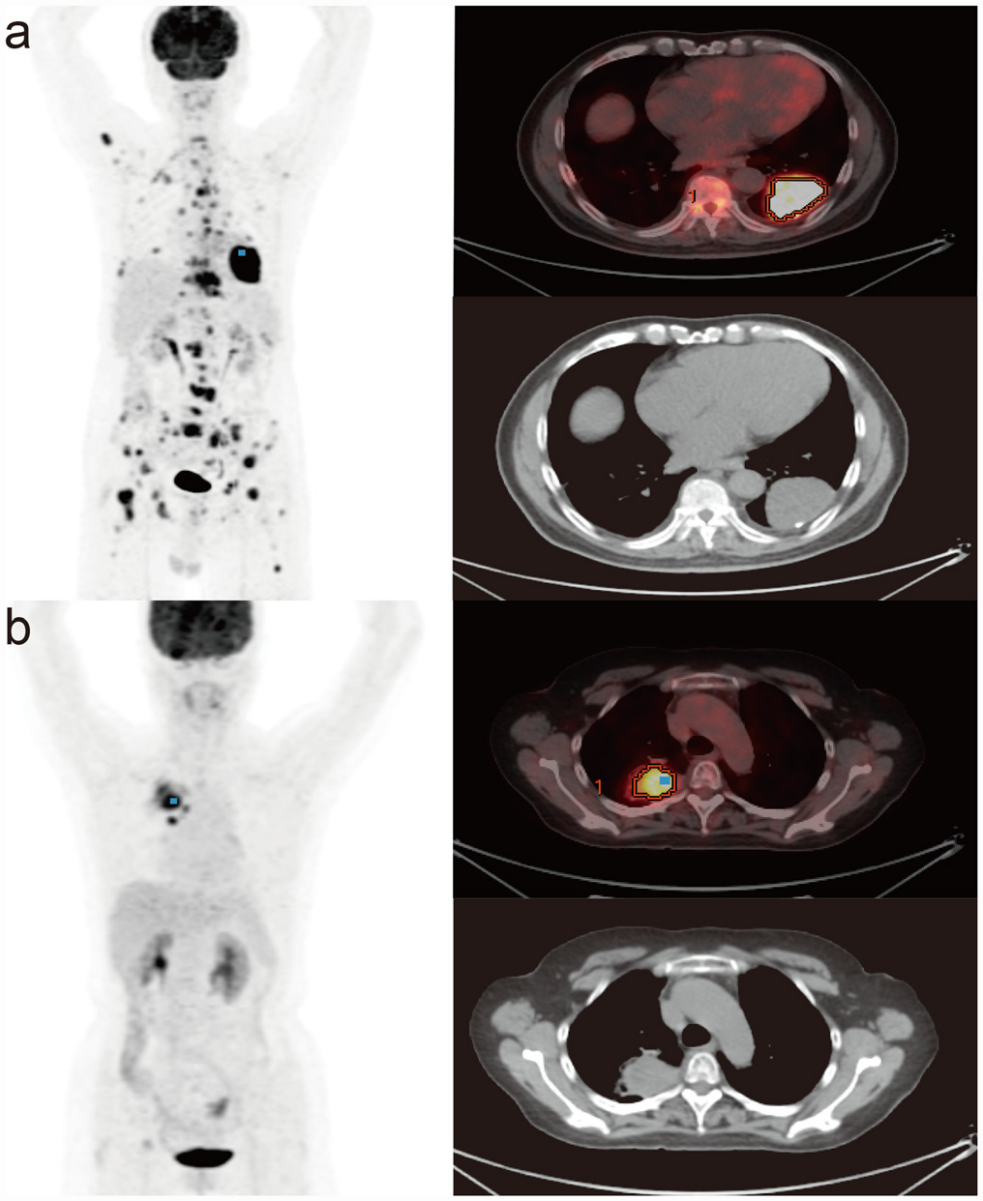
^18^F-FDG PET/CT in patients with advanced lung adenocarcinoma. a A 71-year-old man with high whole-body total lesion glycolysis (TLG_WBR_) determined using the Response Evaluation Criteria In Solid Tumors 1.1 criteria (7944.00) and primary tumor total lesion glycolysis (TLG_T_) (1250.46). Progression-free survival (PFS) was 5.9 months and overall survival (OS) was 11.9 months. EGFR mutation status was the wild-type. b A 68-year-old woman with low TLG_WBR_ (179.74) and TLG_T_ (142.41). PFS was 12.3 months and OS was 35.9 months. EGFR mutation status involved the mutation of exon 19 (delE746-A750).
